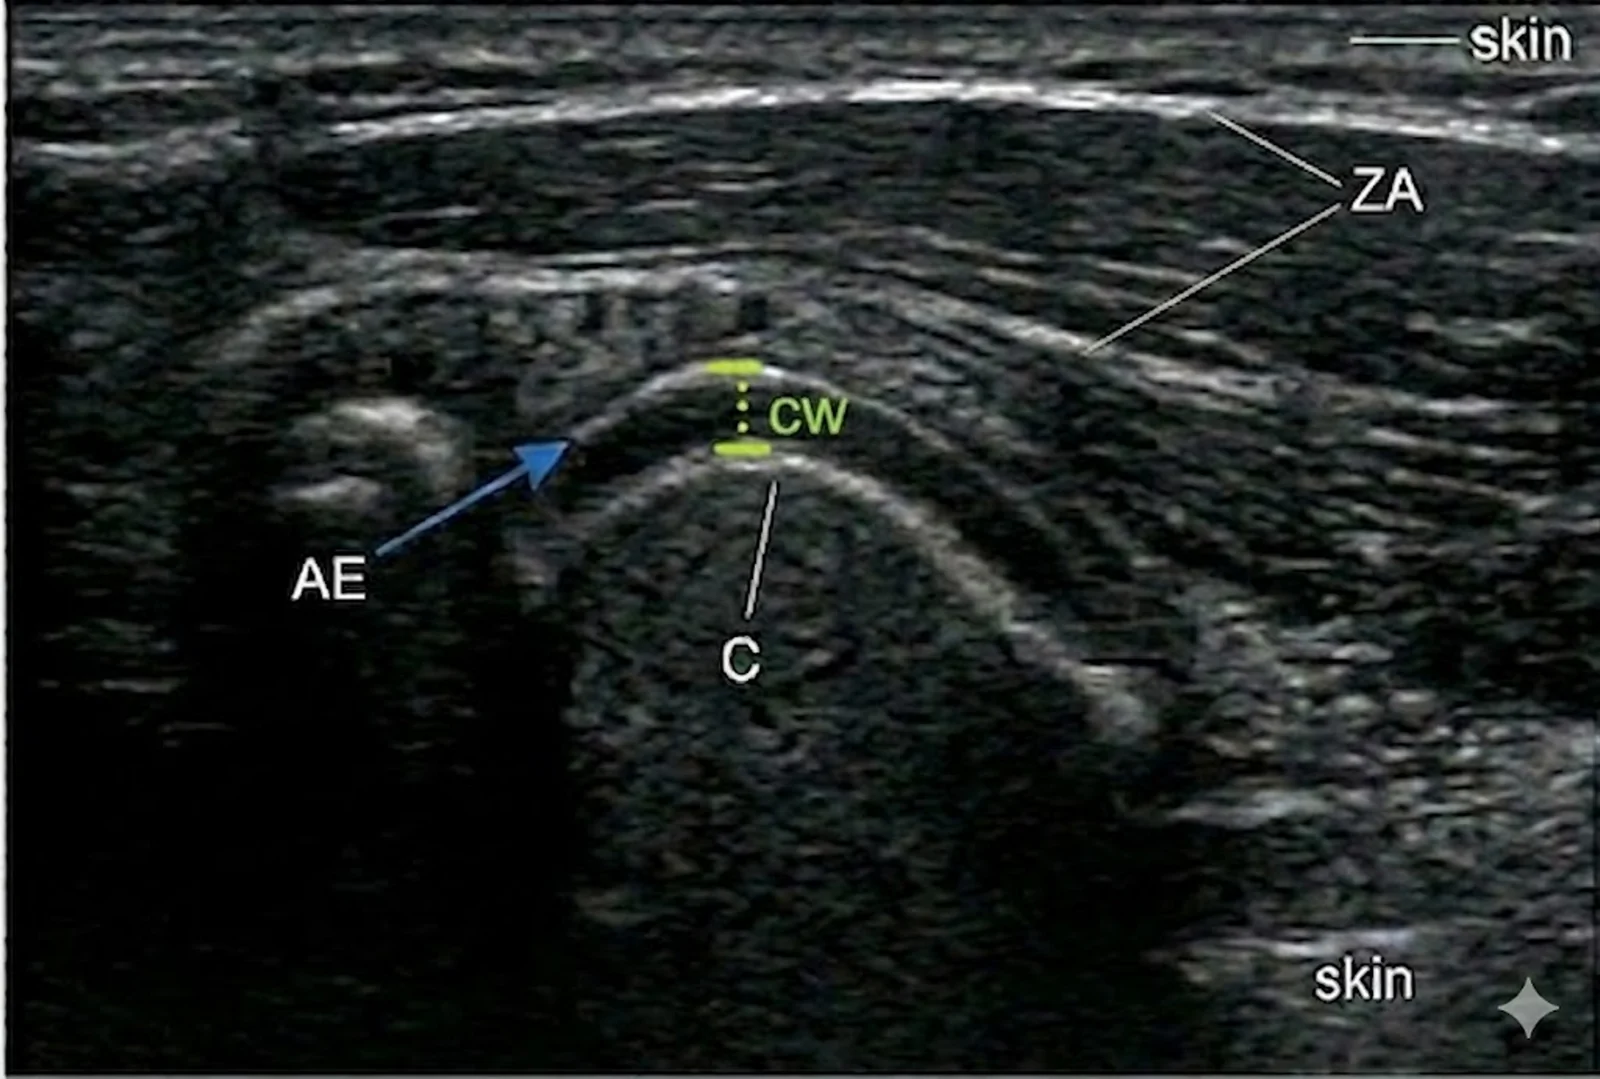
Ultrasonographic evaluation of the temporomandibular joint in the closed-mouth position. The ultrasound image displays the anatomical landmarks and the measurement line: skin surface, ZA (zygomatic arch), AE (articular eminence), and C (hyperechoic cortical outline of the laterosuperior surface of the condyle). The capsular width (CW, indicated by the green dotted line) represents the linear distance measured between the most superior points of the condylar outline and the hyperechoic capsular line
